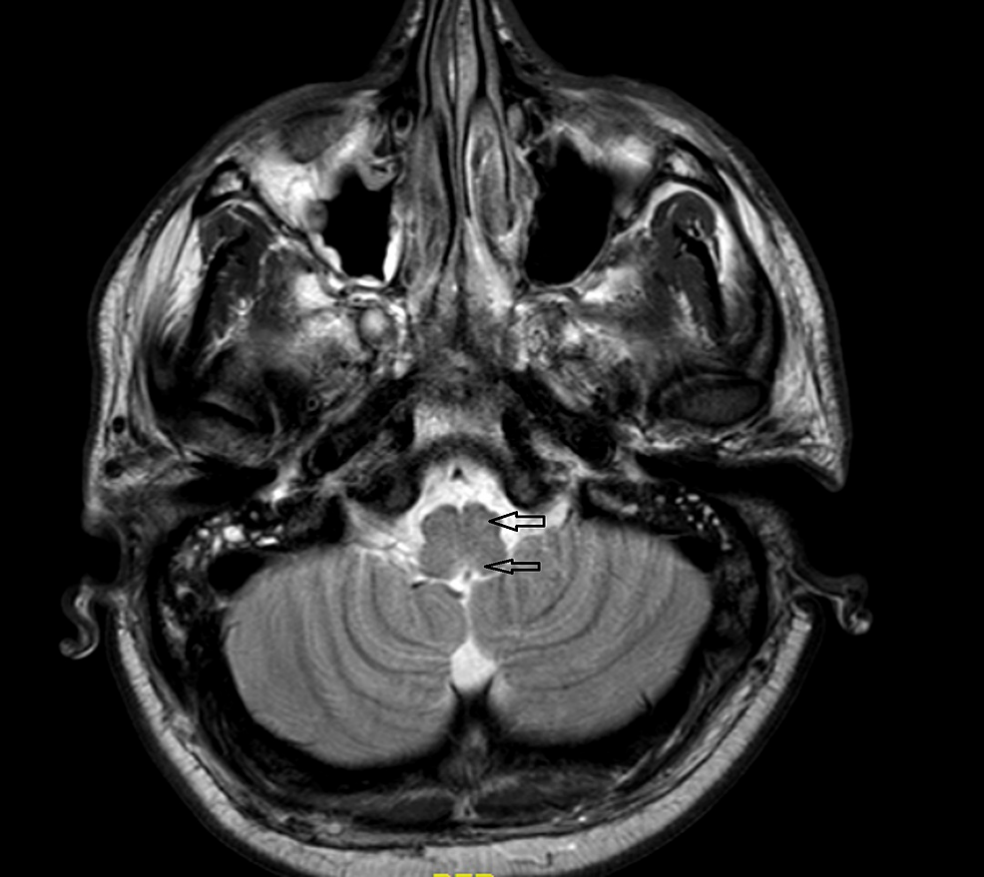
CT scan of cross-sectional view of brainstem showed T2 signal hyperintensity within dorsal brainstem and left cerebral peduncle.
